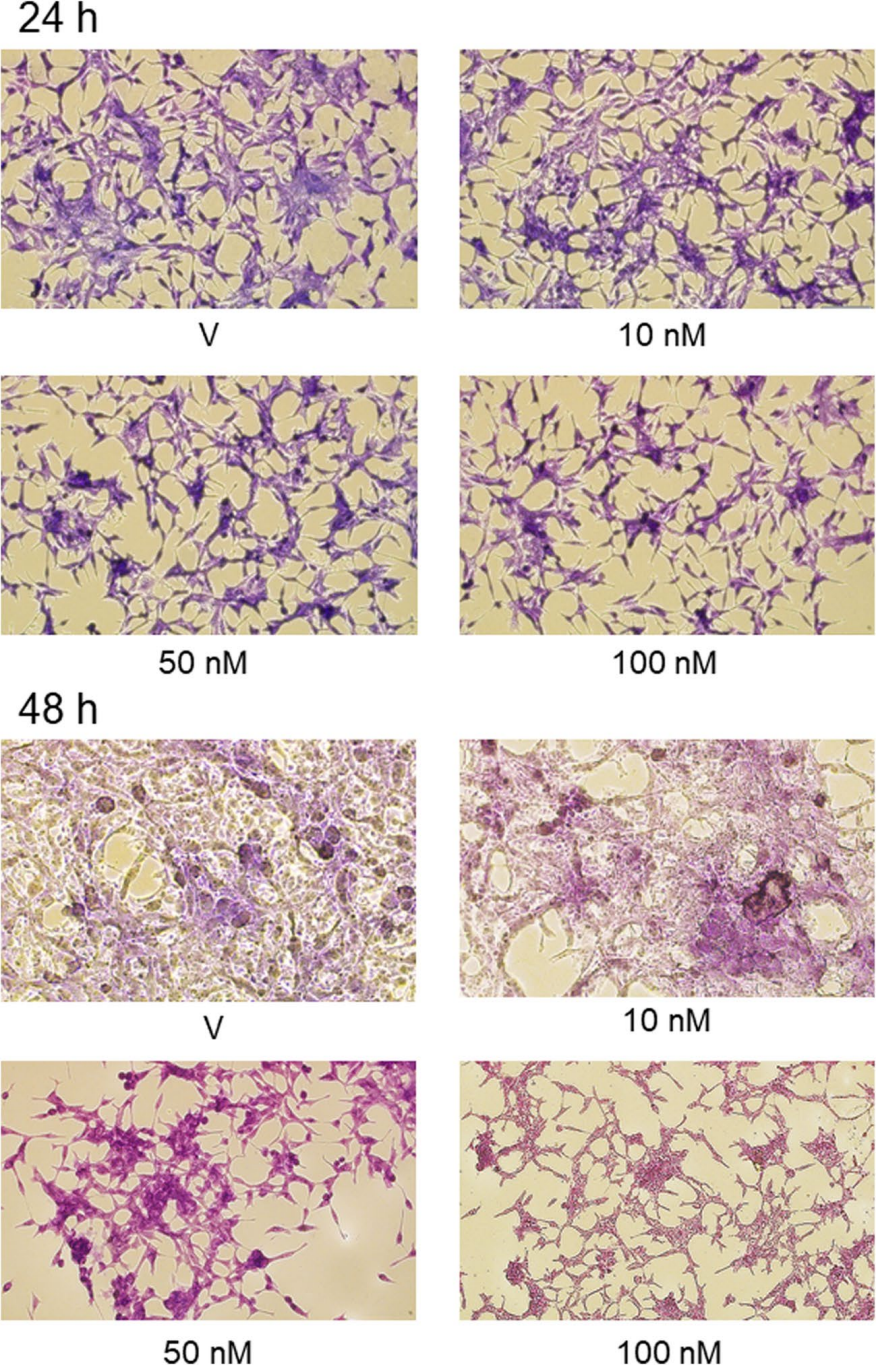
Effects of incubation of LNCaP cell with PSMA-617 for 24 and 48 h on their morphology. LNCaP cells treated with vehicle rapidly proliferate, display irregular size and shape and nuclear atypia, especially after 48 h-lasting incubation. PSMA-617 decreased cell proliferation and, interestingly, the cells acquired an elongated morphology and formed processes (40 × magnification)
